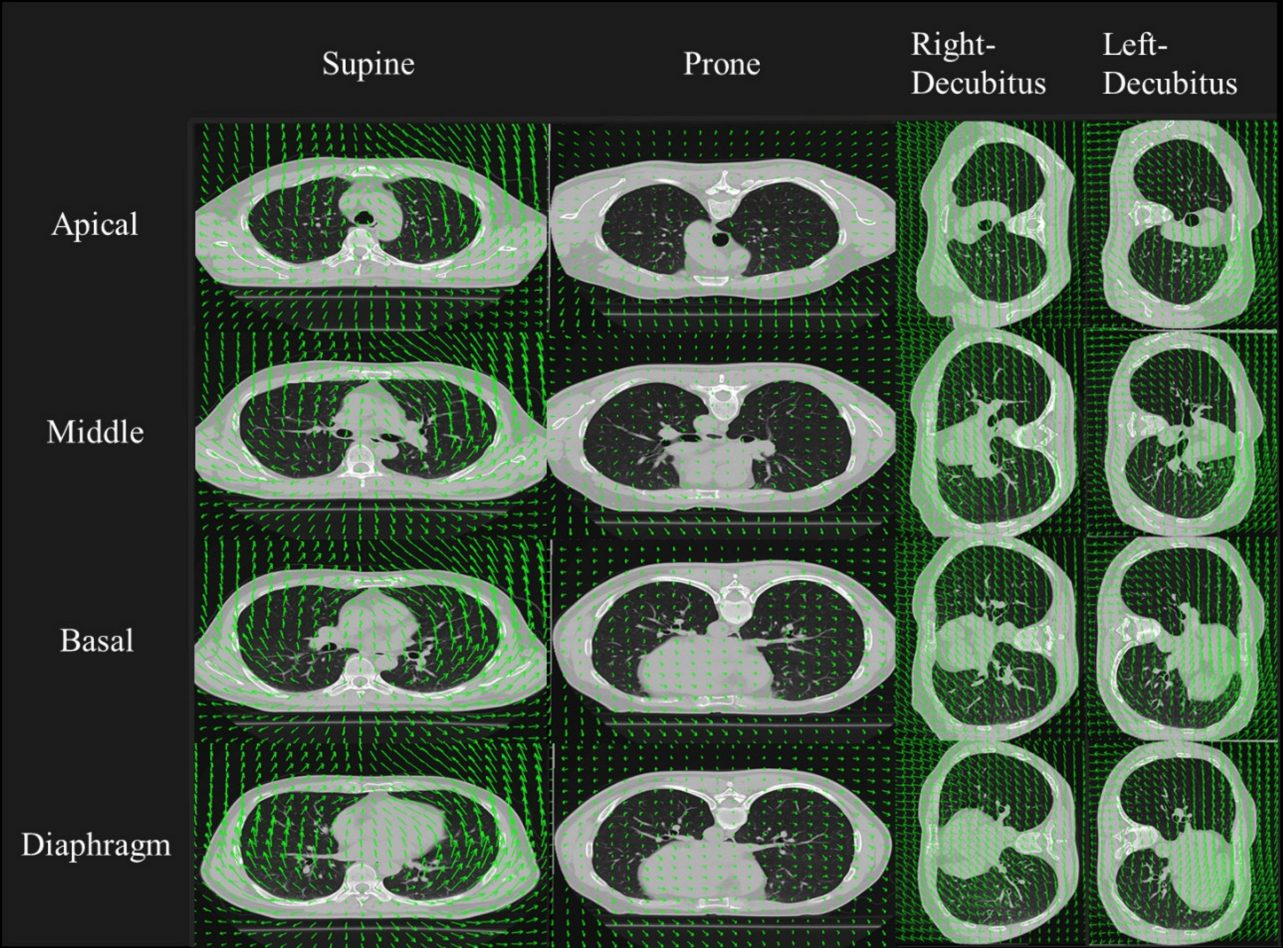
Comparison of deformable vector field (DVF) imaging in each position by deformable image registration (DIR)-based breath hold CT ventilation imaging (BH-CTVI) using expiration and inspiration phase.
